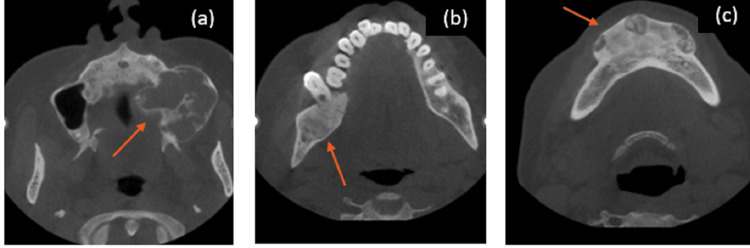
Cone beam CT (CBCT) scans at 12 months post-op CBCT scans at 12 months post-op showing (a) axial section of maxillary lesion revealing radiolucency with radiopaque flecks suggestive of osteoid formation, (b) axial section of posterior mandibular lesion showing complete opacification of the lesion along with resolution of the breach in the lingual cortex, and (c) axial section of the mandible showing opacification of the symphyseal lesion.
